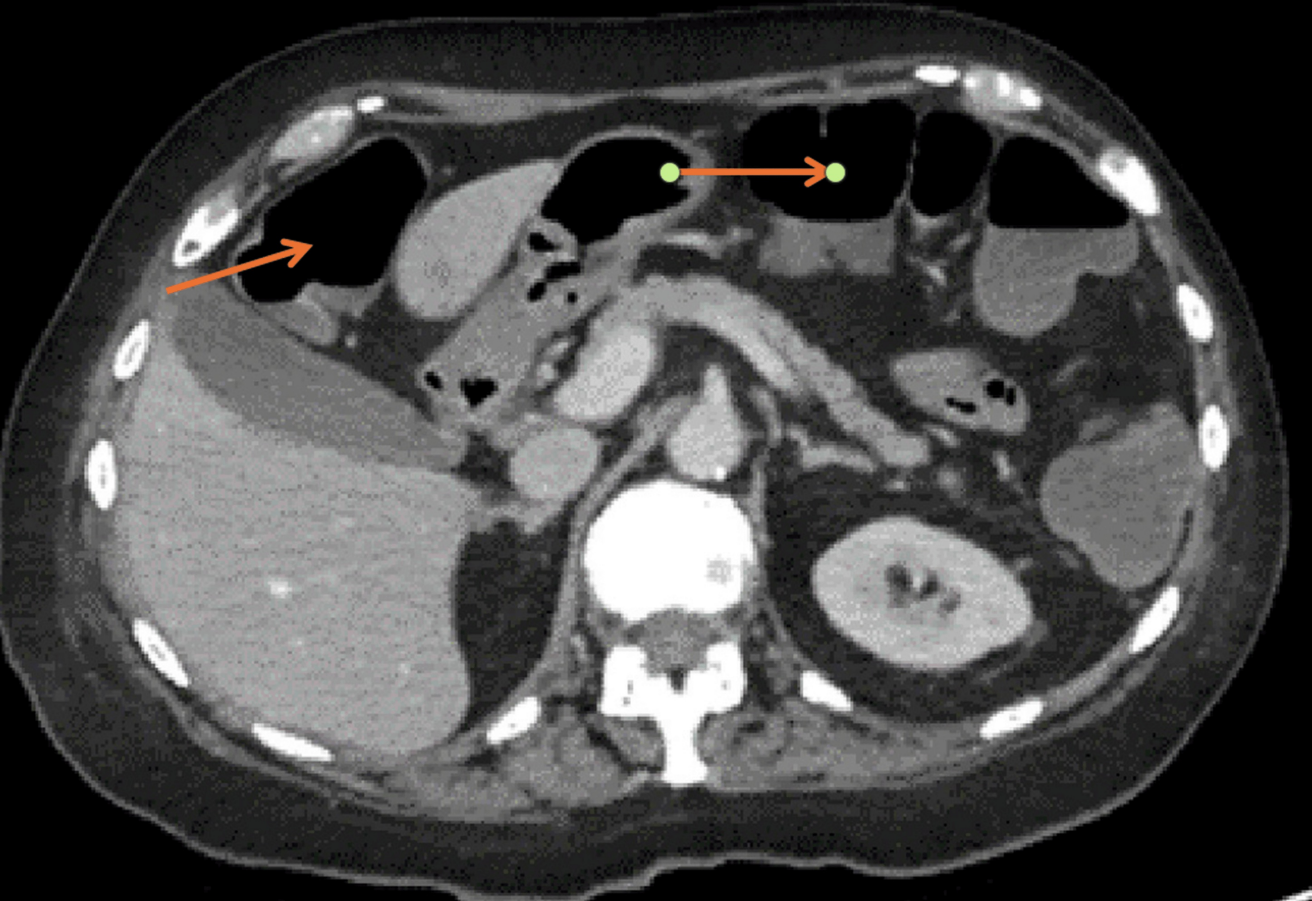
CT abdomen axial view showing bowel dilatation (arrows).
